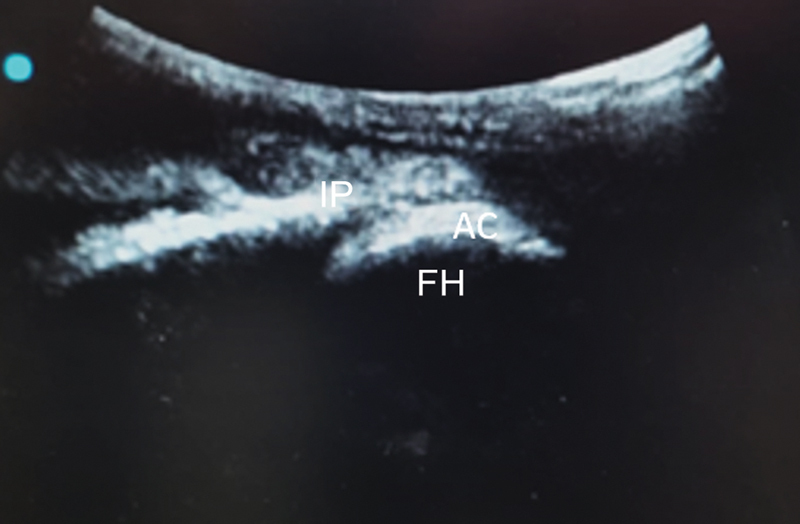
Ultrasound view of the “stacking sign” with the transducer longitudinal to the frontal plane.
